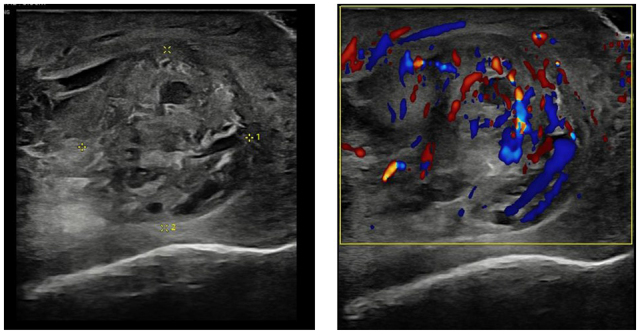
Ultrasound of the left testis showing epididymitis with a small complex fluid collection along the distal epididymis raising suspicion for an abscess.
